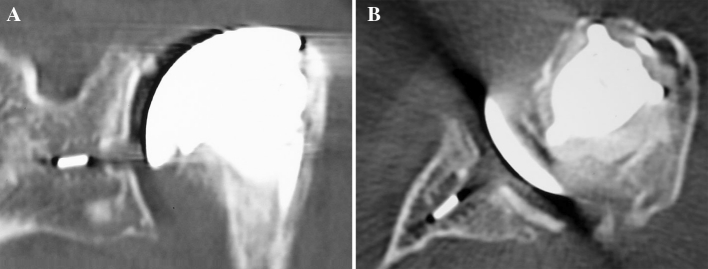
CT scan evaluation: bone ingrowth between the flanges of the central peg
